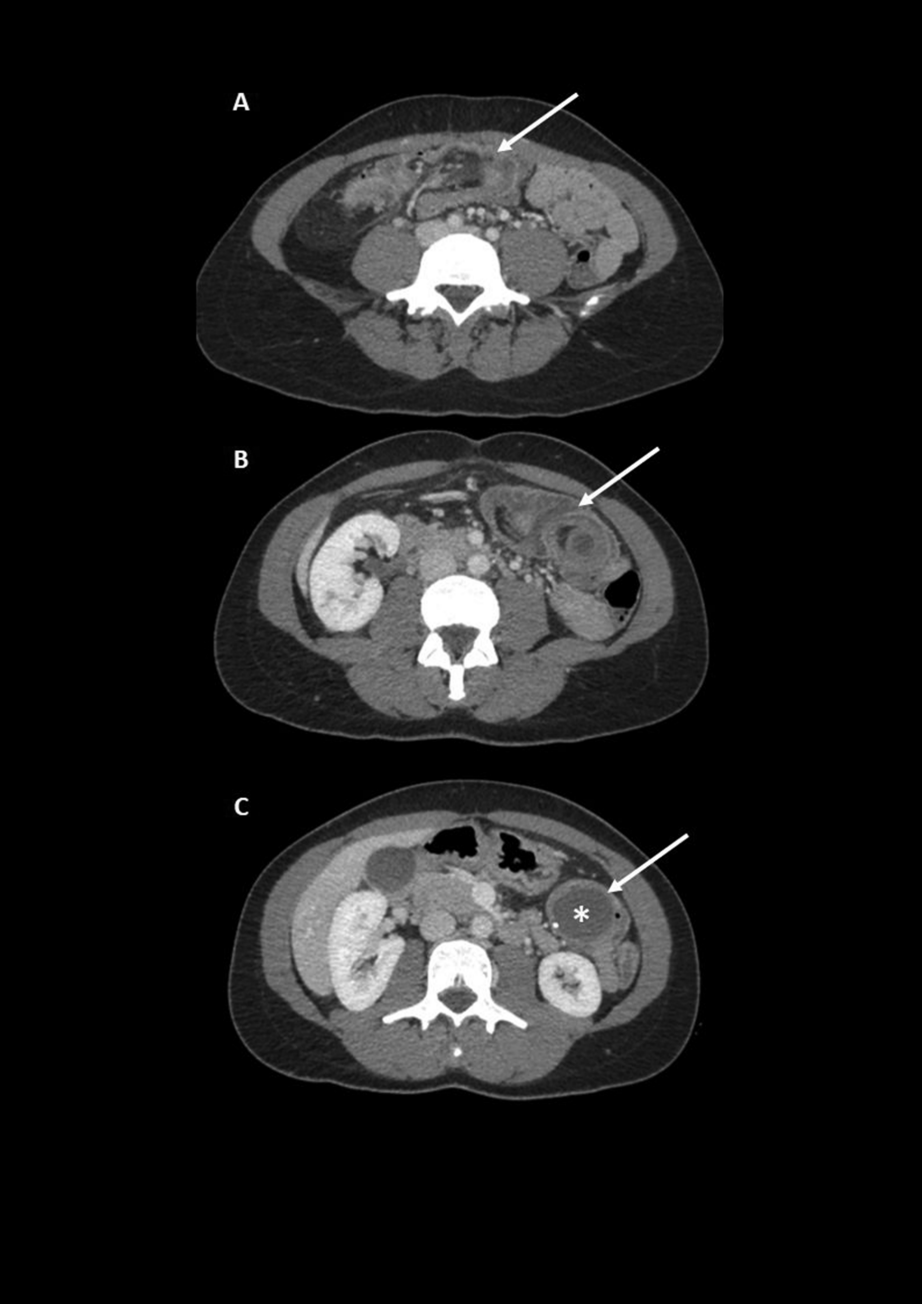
Abdominal CT scan revealed an ileo-caecal intussusception through the left transverse colon (panels A, B with a target sign, arrow) caused by an appendicular mucocele filled with mucin, presenting parietal calcification (panel C, arrow).
